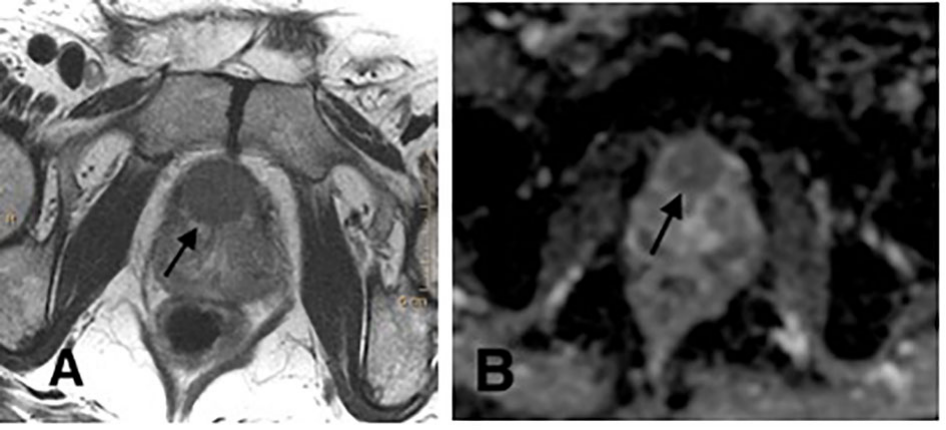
(A) Axial T2-weighted image with a hypointense lesion on the anterior transitional zone of the middle third bilaterally. (B) Axial diffusion-weighted image (ADC maps) with a marked diffusion restriction on the same zone. PI-RADS 5.
